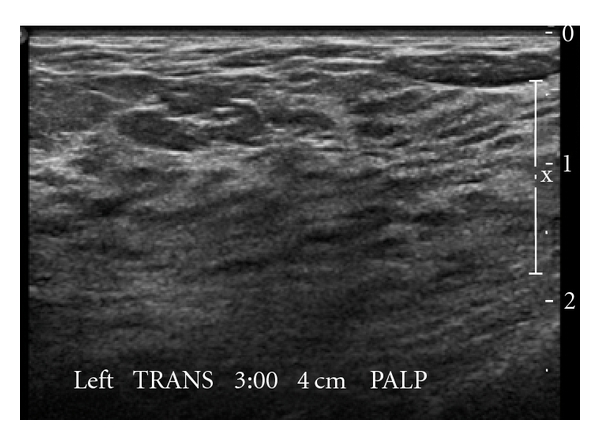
Ultrasound of the palpable abnormality in the left breast shows normal tissue with no mass.
